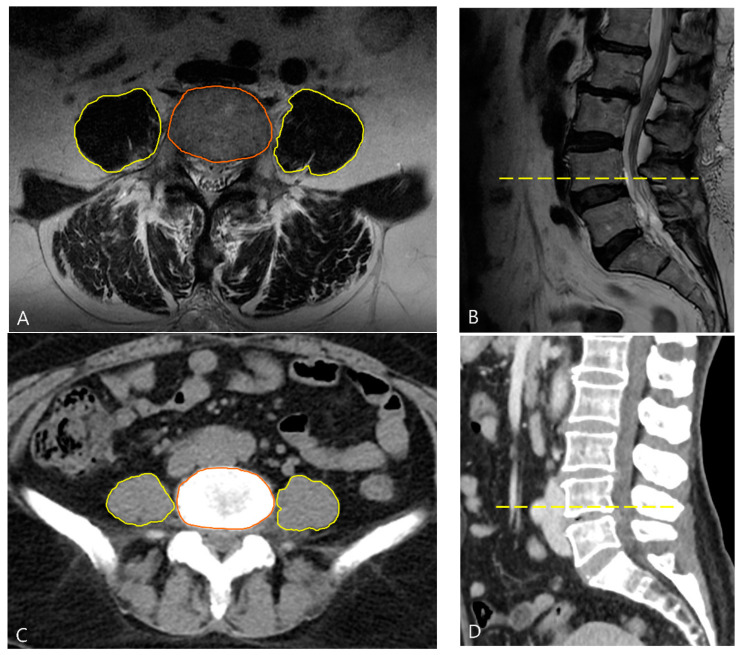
Representative cross-sectional (A) and sagittal (B) MRI images. Measurement of the right and left psoas muscle areas (outlined in yellow) and vertebral body area (outlined in orange) at the L4 level. Cross-sectional image was obtained at the level of the dashed line in (B). (C,D) Representative cross-sectional and sagittal abdominopelvic CT images, respectively. Measurement of the right and left psoas muscle areas (outlined in yellow) and vertebral body area (outlined in orange) at the L4 level. Cross-sectional image was obtained at the level of the dashed line in (D). CT, computed tomography; MRI, magnetic resonance imaging.
